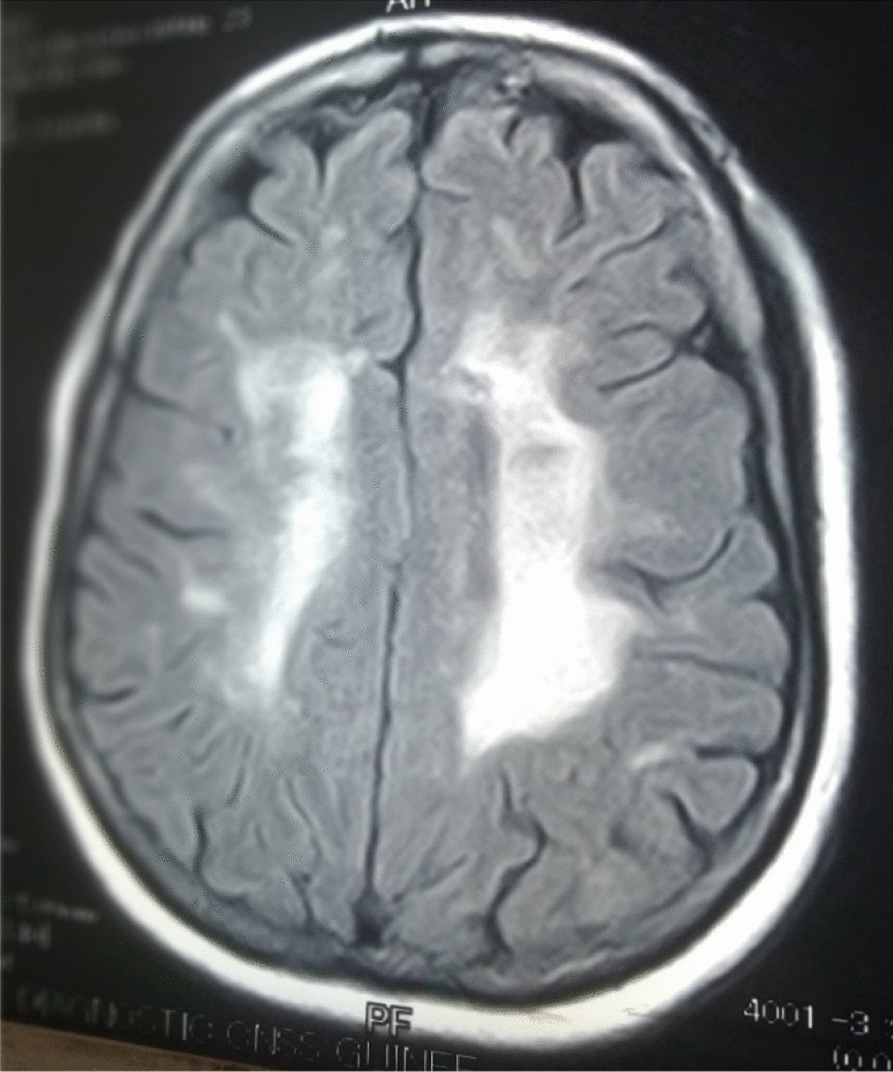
Cerebral MRI: focal abnormality in the white matter periventricular hyperintensities
